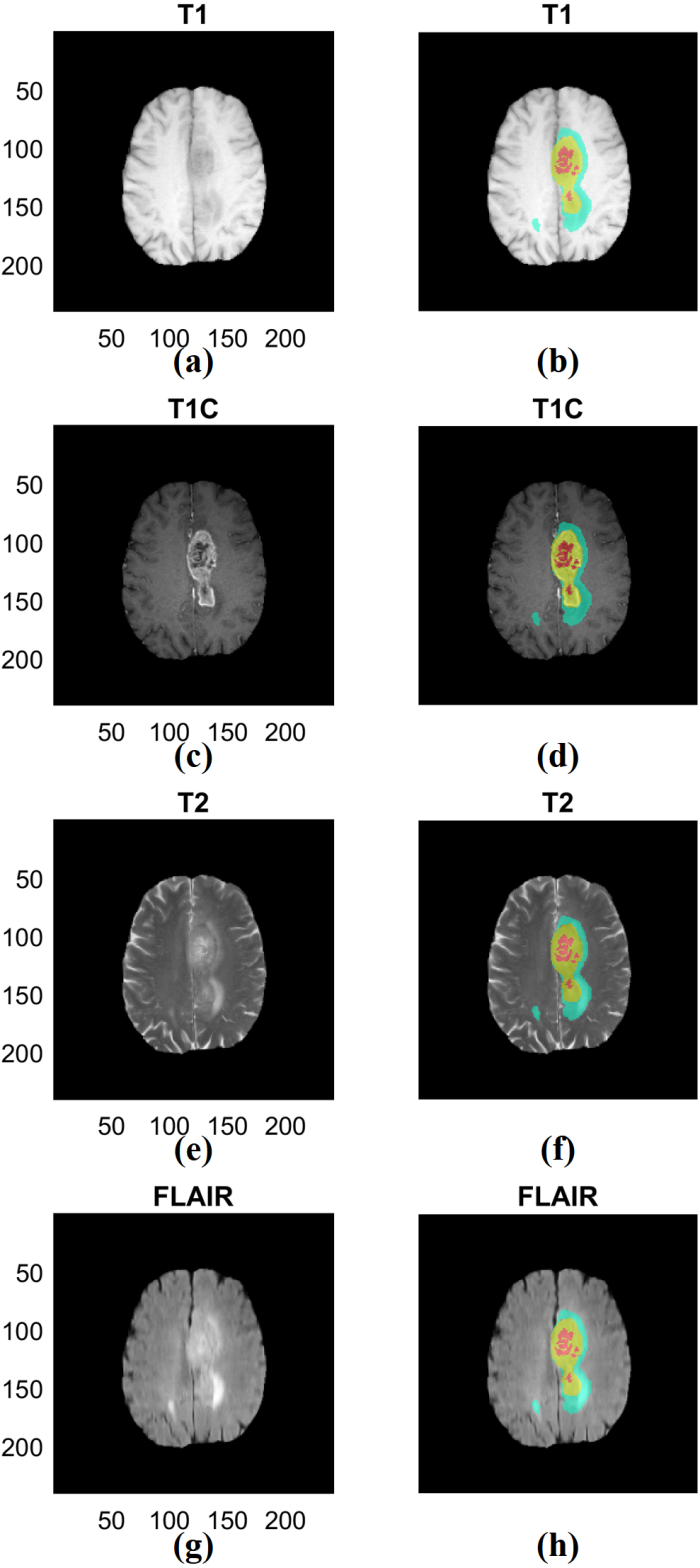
Examples of three types of ROIs used in our study. (A) T1 data. (B) ROI associated with T1. (C) T1C data. (D) ROI associated with T1C. (E) T2 data. (F) ROI associated with T2. (G) FLAIR data. (H) ROI associated with FLAIR. The left column (A) (C) (E) (G) shows different imaging modalities. The right column (B) (D) (F) (H) shows associated ROIs. The ROIs were specified in 3D but 2D representative examples are given. ROIs are visualized in the right column. Red indicates non-enhancing tumor and necrosis (ROI type I), yellow indicates enhancing tumor (ROI type II) and blue indicates edema (ROI type III) in the right column. T1; T1-weighted image, T2; T1C; T1-contrast enhanced; T2-weighted image, FLAIR; Fluid-Attenuated Inversion Recovery.
